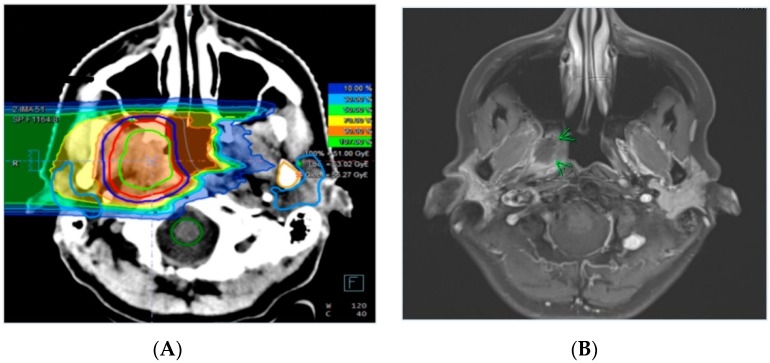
Carbon ion re-irradiation treatment plan of a patient with a recurrent adenoic cystic carcinoma (ACC) of the right nasopharyngeal wall treated with 51 Gy (RBE) in 17 fractions (A). Corresponding axial contrast-enhanced T1-weighted MR sequence of the same patient prior to re-irradiation (B).
